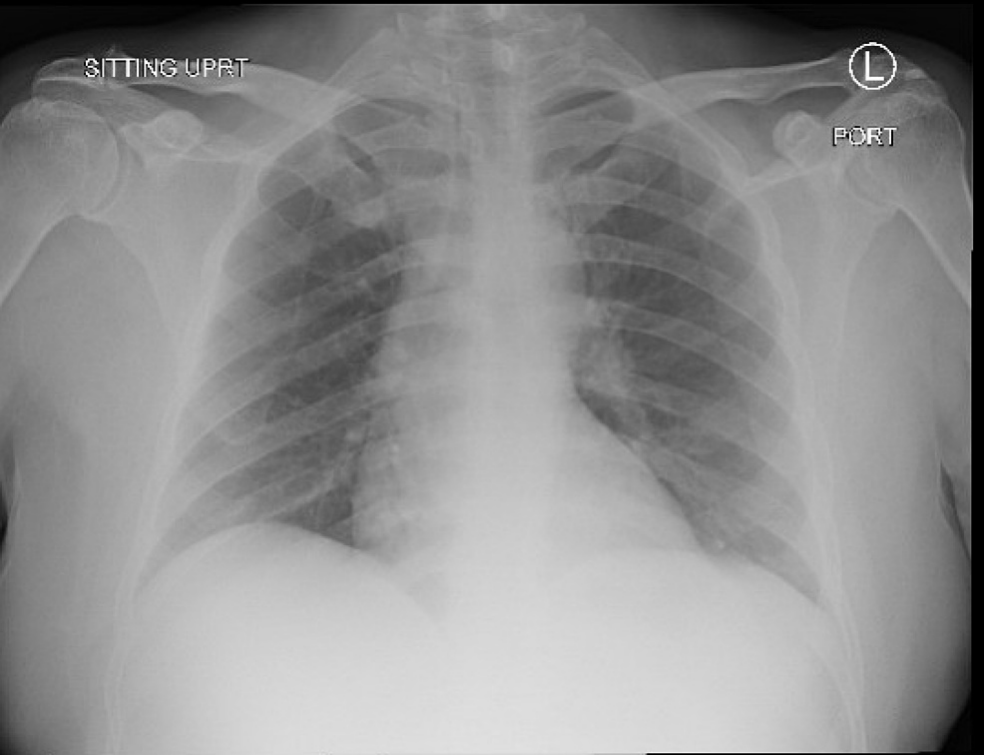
Initial chest radiograph showing no focal evidence of airspace disease
